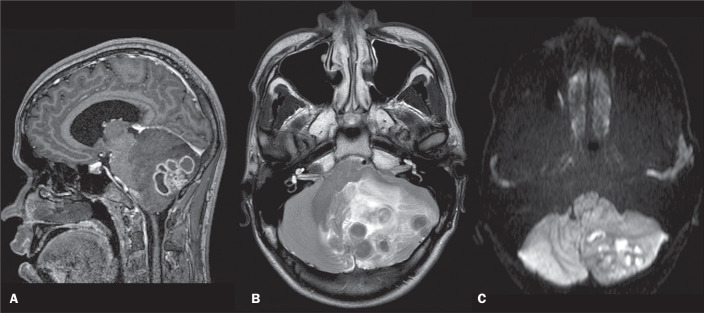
Peripheral ring-enhancing lesions on a contrast-enhanced sagittal T1-weighted image (A). Lesions showing hypointense signal, together with perilesional edema, on a T2-weighted image (B) and marked restricted diffusion on diffusion-weighted imaging (C). The lesions also showed hypointense signal on T1-weighted imaging and on susceptibility-weighted imaging (not shown). The final diagnostic was tuberculosis.
